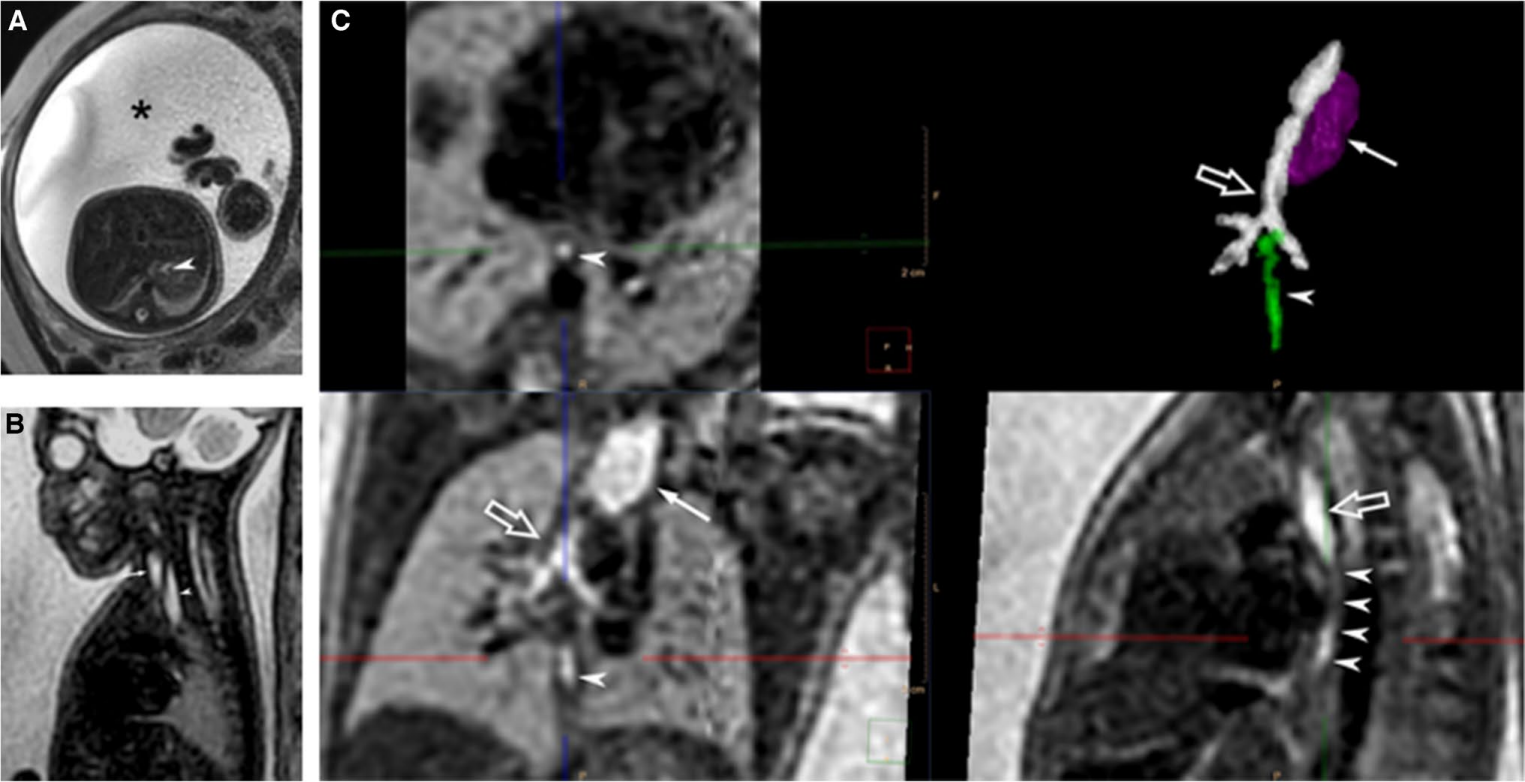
T2-weighted single-shot fast spin echo (SS-FSE) fetal MRI performed at 30 2/7 weeks using a 1.5-T scanner (Philips Achieva dStream, Philips Healthcare, Amsterdam, The Netherlands). A Axial image at the level of the upper abdomen shows polyhydramnios (*asterisk*) and small stomach (*arrowhead*). B Midline sagittal view of the chest shows a dilated upper esophageal pouch (*arrowhead*) posterior to the trachea (*arrow*). C Super-resolution SVR images of the fetal chest displayed in orthogonal axial, coronal, and sagittal planes. The images were manually segmented to produce the 3D-rendered image of the esophageal atresia with distal fistula. The atretic esophageal segment resulted in a dilated upper esophageal pouch (*arrows*) which was segmented out and colored *dark magenta*, the trachea and bronchi were segmented in *white* (*open arrows*), and the distal esophagus fistulized from the proximal left main stem bronchus (*arrowheads*) is colored *green*
